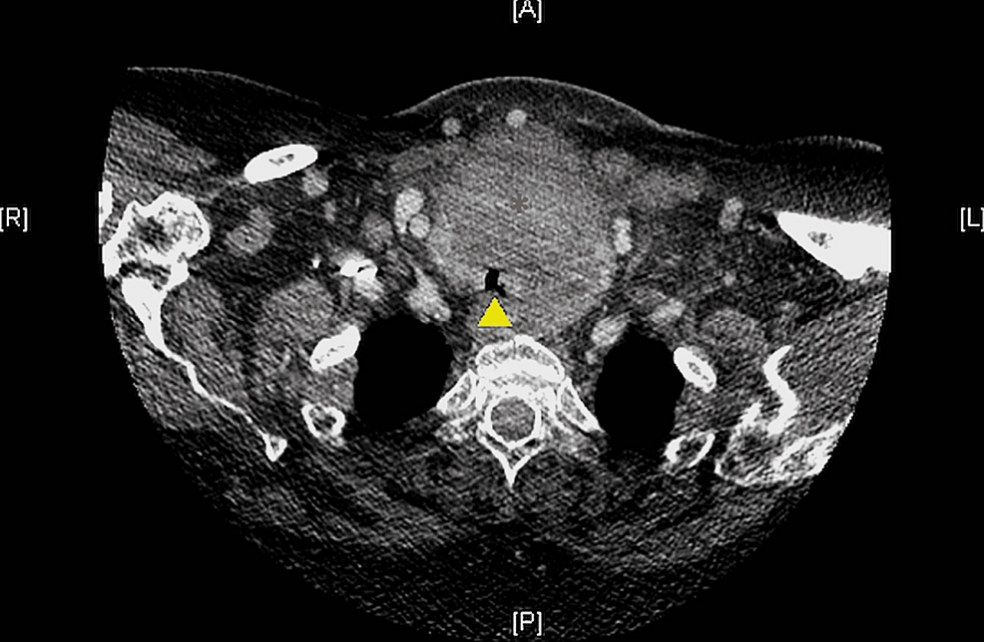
Coronal CT image of the chest at the T1 vertebral level Compression of the tracheal lumen (yellow arrow tip) by the mediastinal mass at its narrowest point can be seen.
